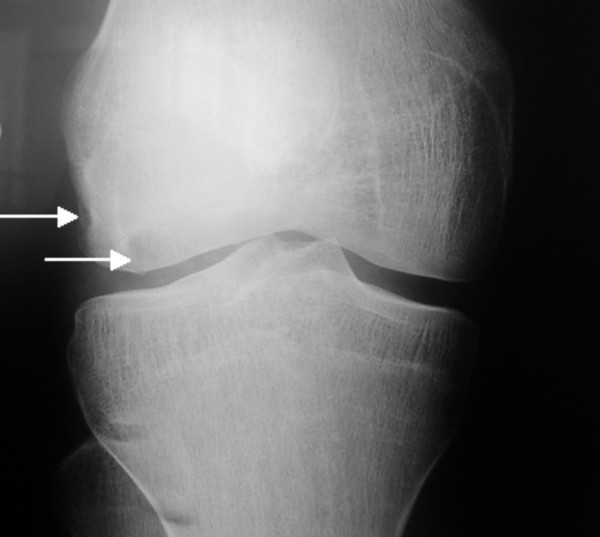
Patient 1: Anteroposterior knee radiograph at age of 15 years shows osteochondritis dissecans of the distal femoral epiphysis with areas of evident epiphyseal necrosis (arrows).
